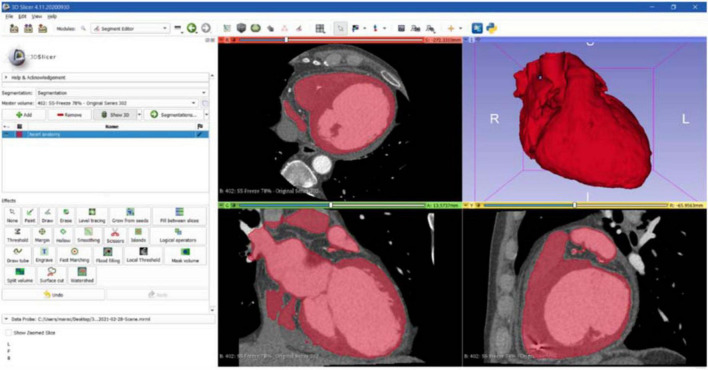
CT segmentation. Axial, sagittal, coronal, and 3D views of CT segmentation on the 3D Slicer software.
